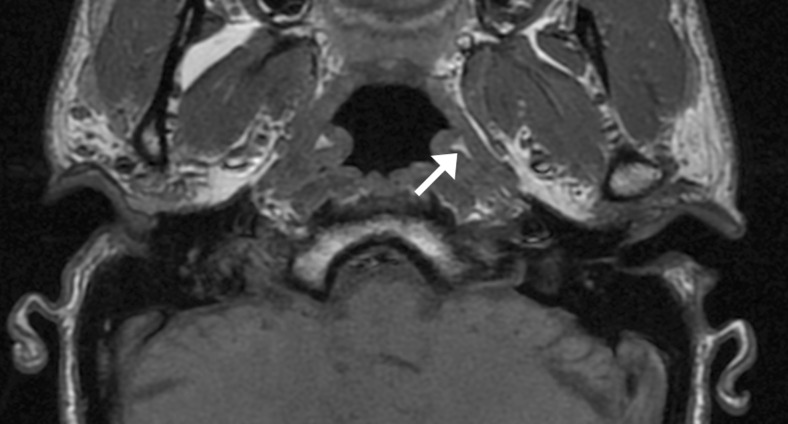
Axial T1 spin echo MRI of the nasopharynx in a 36-year-old healthy male volunteer. Ostmann fatty tissue is indicated on the left by the *arrow*
